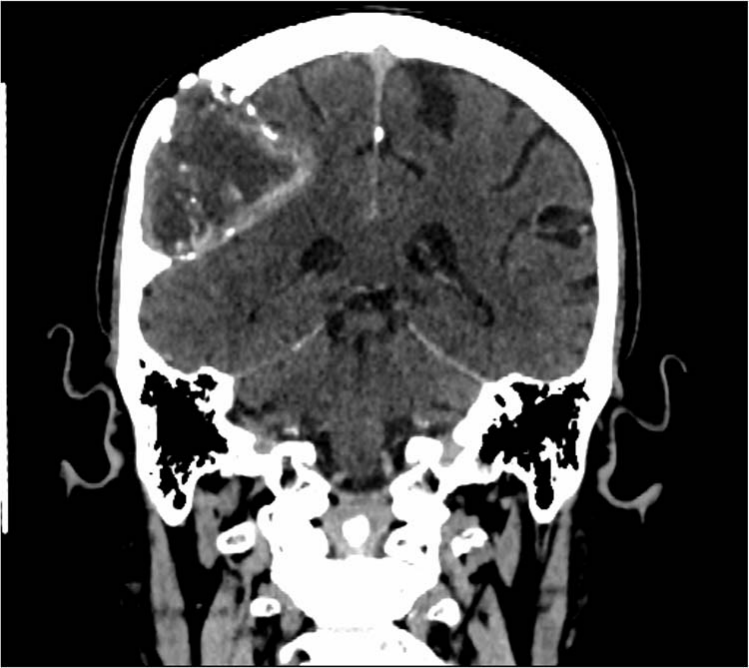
Sagittal CT scan showing the extra-axial lesion with surrounding bone remodelling and evidence of the bone erosion as well.
